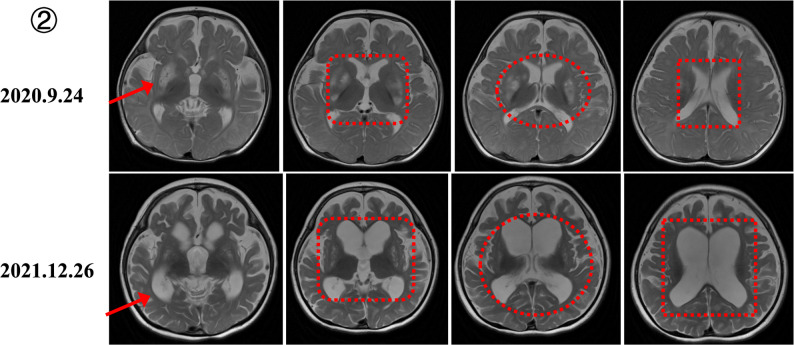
MRI progression in LS due to MT-ATP6 m.8993T > G mutation (2020–2021). Images demonstrate progressive ventricular enlargement, persistent basal ganglia lesions, and evolving cortical atrophy during 15 months of follow-up
